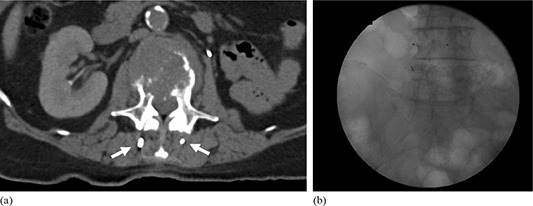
Images from patient who presented with painful bony metastases to the fourth lumbar vertebral body with three RF transmitter devices surgically implanted into the paraspinal muscle for single‐fraction stereotactic body radiotherapy for pain relief and quality of life enhancement. RF transmitters imaged on (a) computed tomography and (b) a c‐arm fluoroscopic system.
